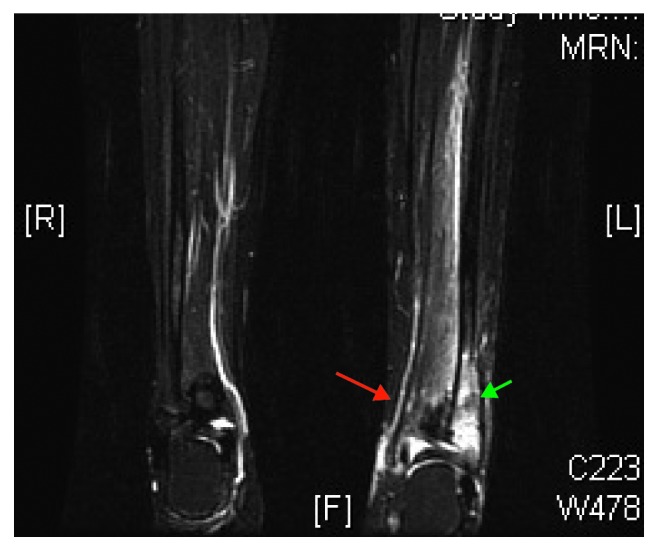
MRI of distal left fibula (green arrow) and tibia (red arrow) showing multifocal chronic osteomyelitis.
